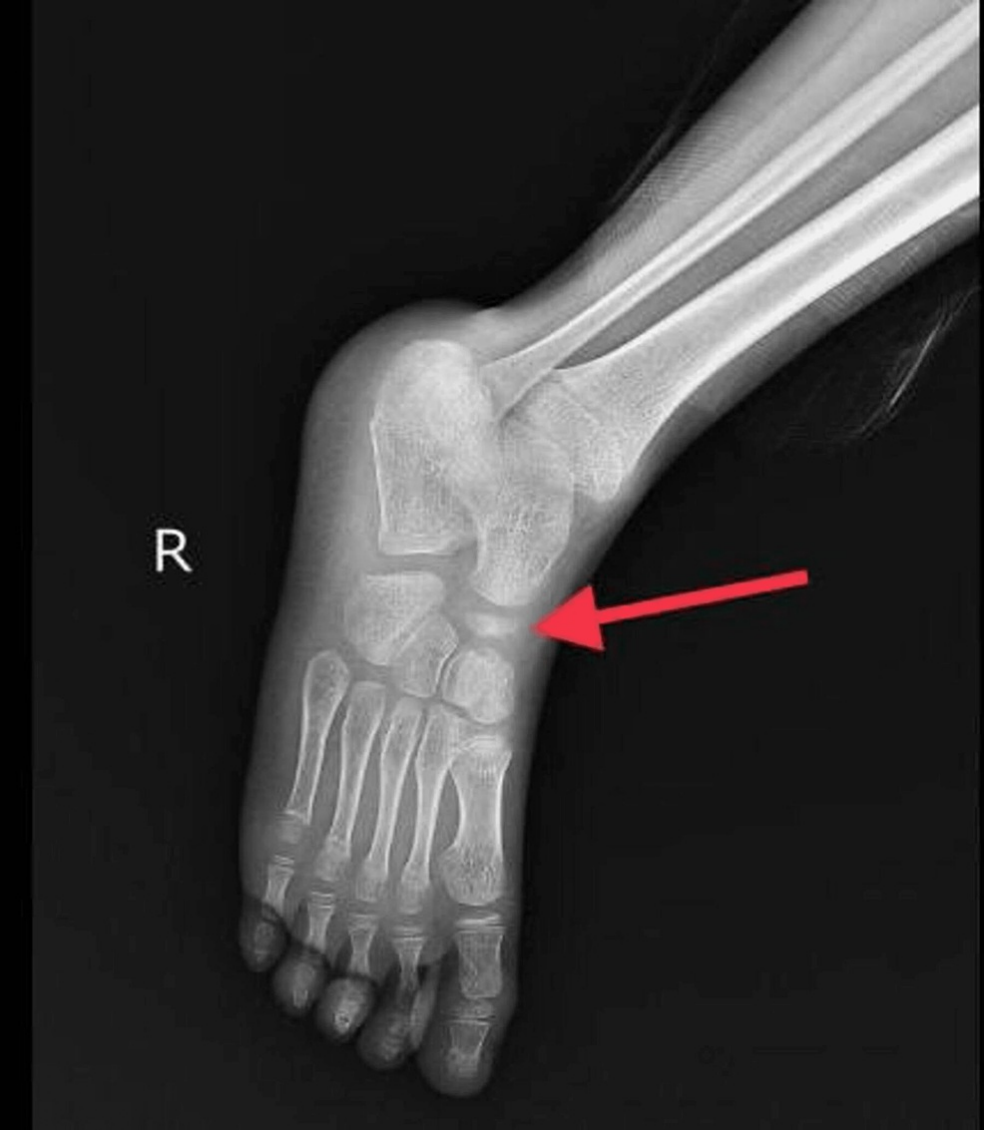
X-ray oblique view of the right foot and ankle
